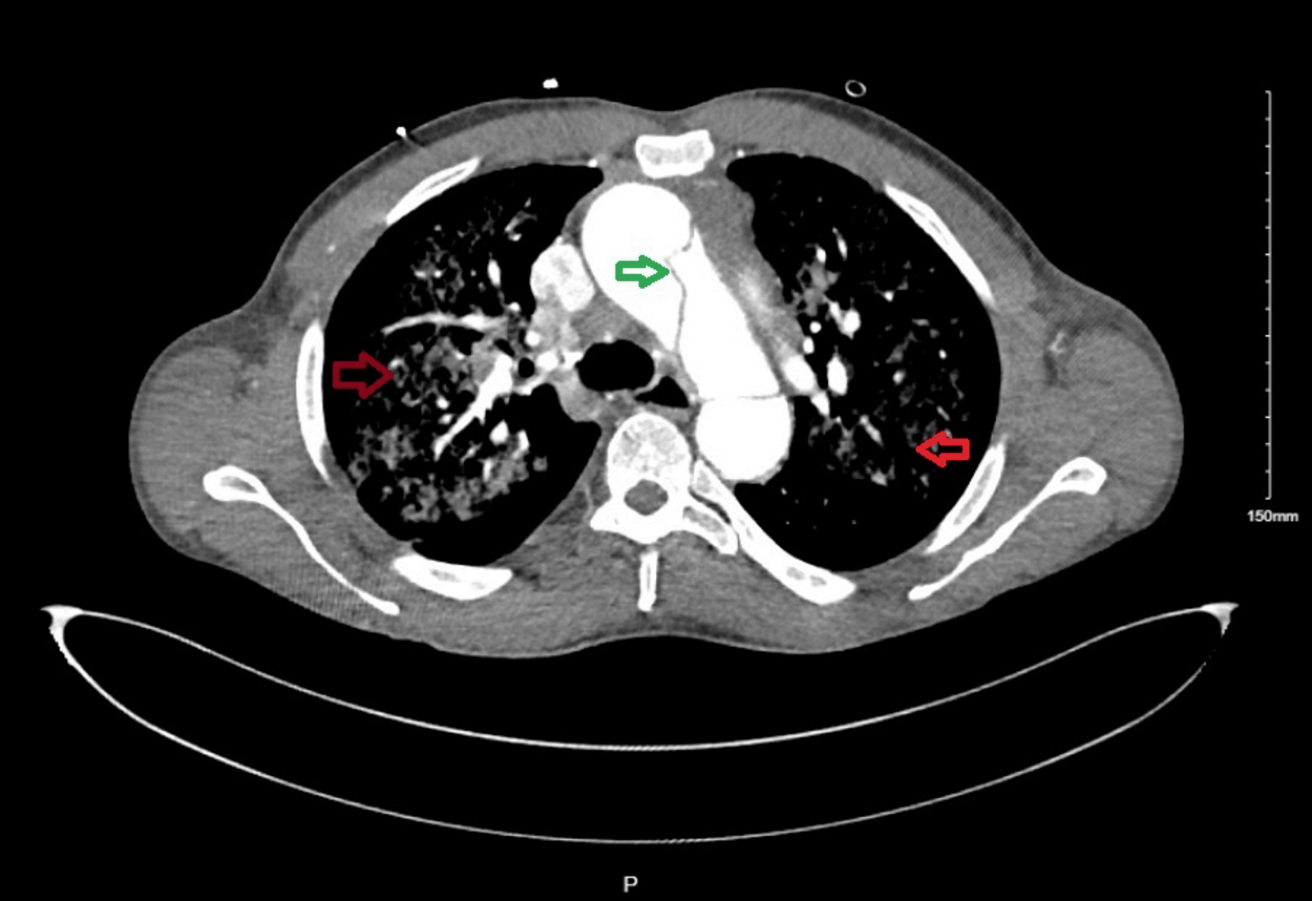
CTA of the chest - transverse plane Green arrow - acute type A aortic dissection involving the ascending aorta extending into the left subclavian artery and descending aorta. Red arrows - extensive ground glass opacities present in all lobes of the lungs CTA: computed tomography angiography
